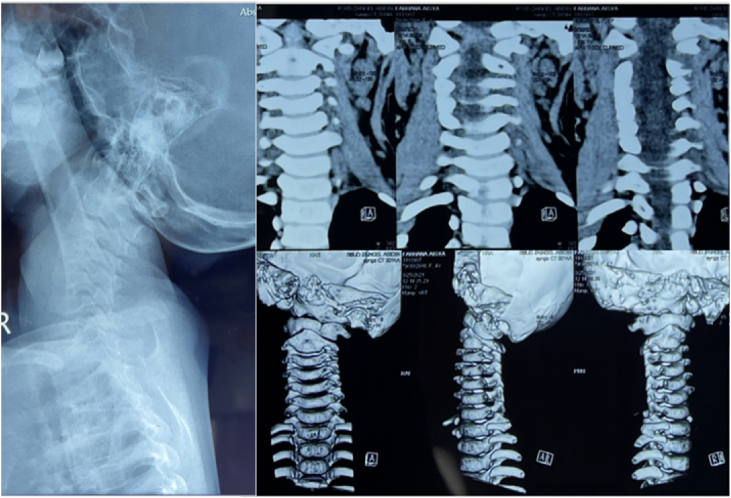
Cervical plain radiograph and cervical spine CT-Scan.
